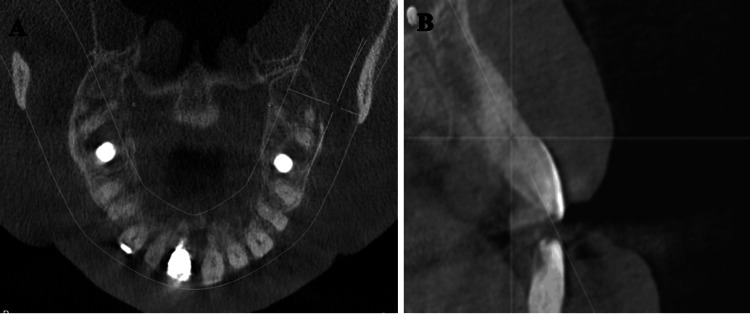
CT scan A. Axial and B. Lateral view of CT scan of the upper jaw, which showed unremarkable findings in bone volume and density
